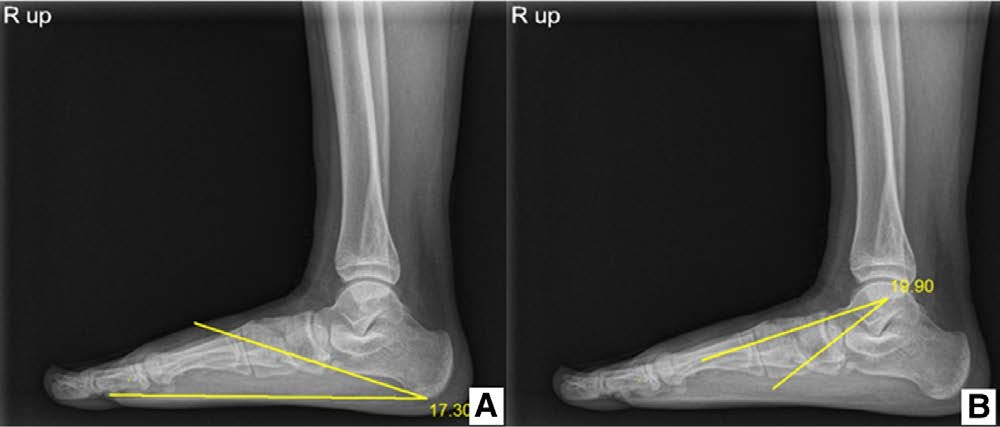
(A) Calcaneal pitch angle (CPA) and (B) Meary angle (talo-first metatarsal angle) (TMA) by standing lateral radiographs of foot. CPA = calcaneal pitch angle, TMA = talo first metatarsal angle.
